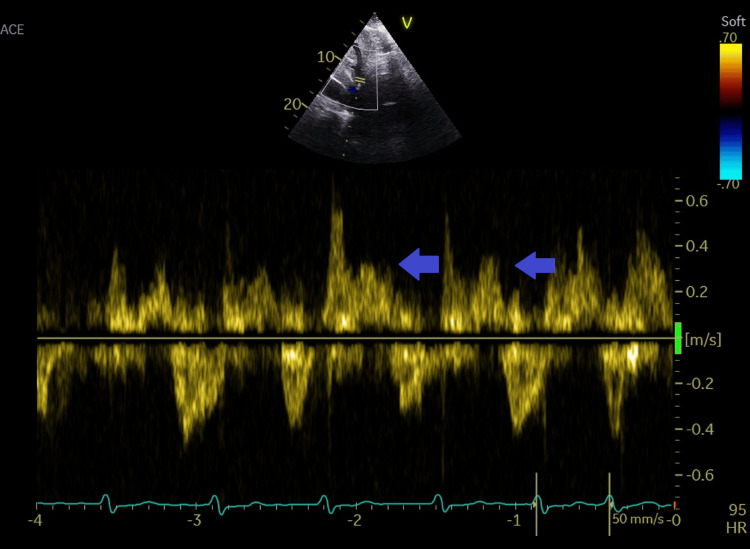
Pulsed wave Doppler recording in the hepatic vein showing systolic reversal of flow, indicating severe tricuspid regurgitation. Blue arrows show systolic reversal spectral display
